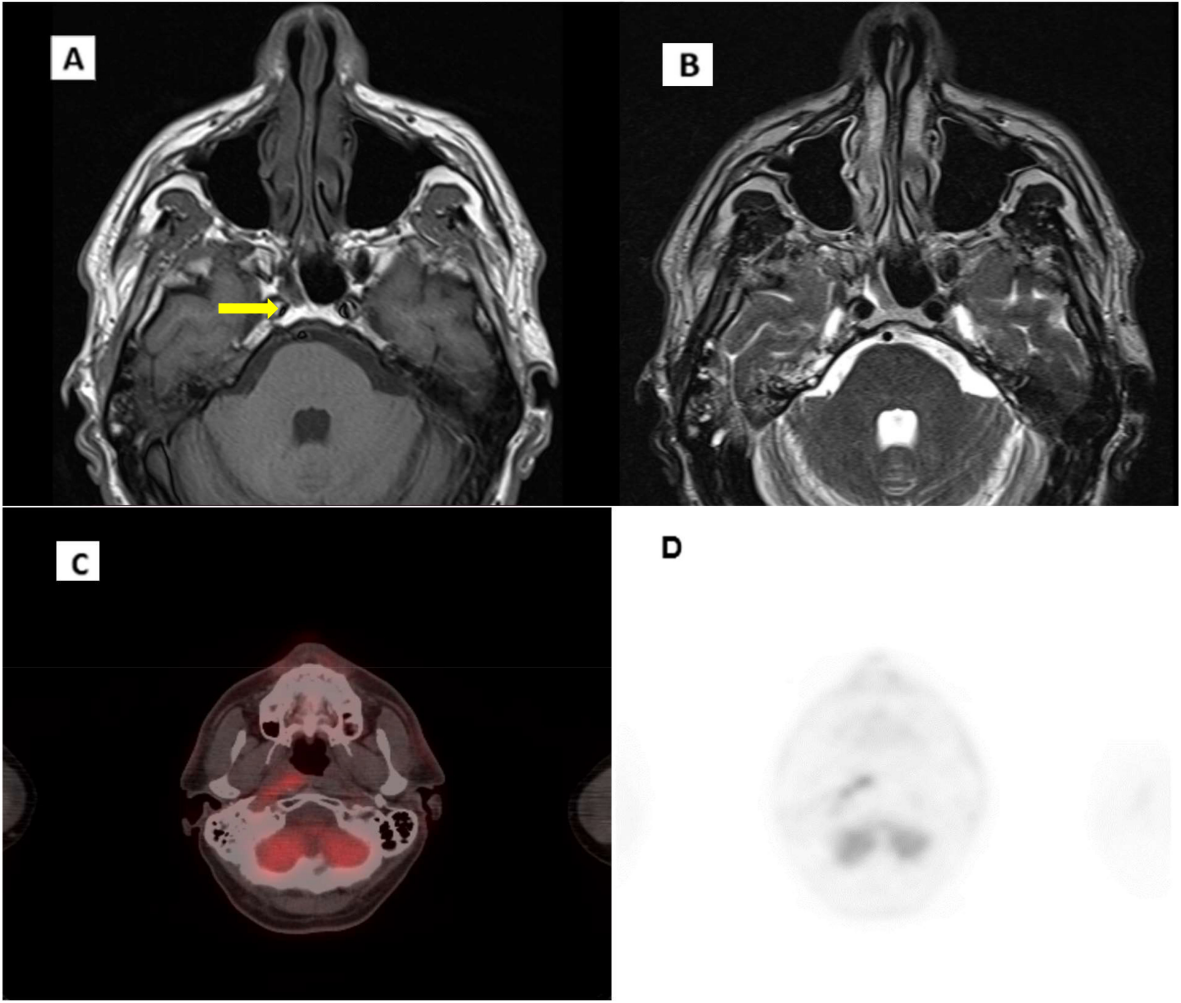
Evidence of disease recurrence. (A) T1 MRI axial scan. (B) T2 axial scan. (C), (D) CT/PET axial scan. Arrow indicates pathological thickening of the nasopharynx.
